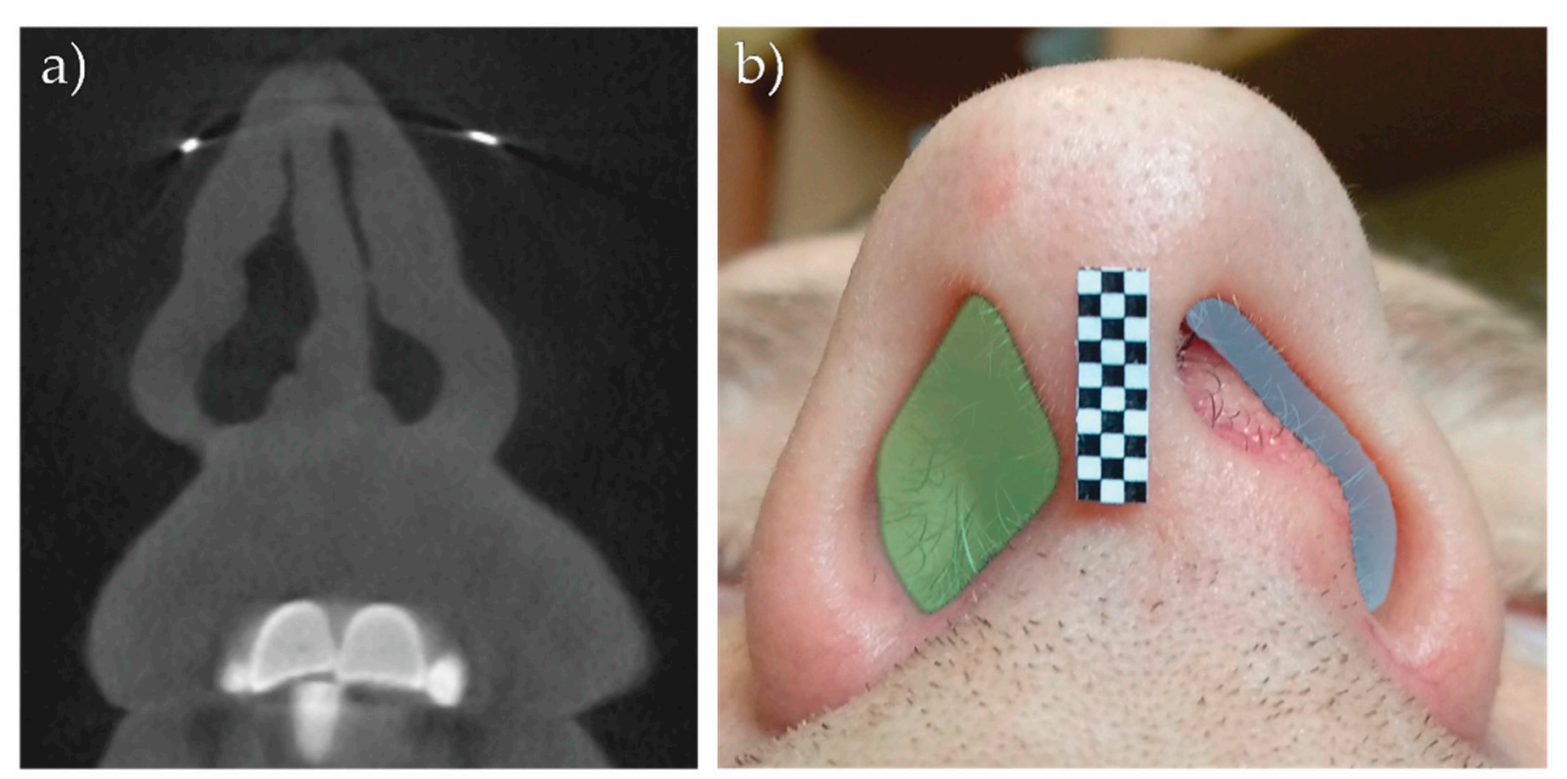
(a) Computed Tomography scan of a patient with deviated septum nasal; (b) photo with marked nostrils surface (light blue 25 mm^2^, light green 69 mm^2^, marker with 1 × 1 mm measure reference).
